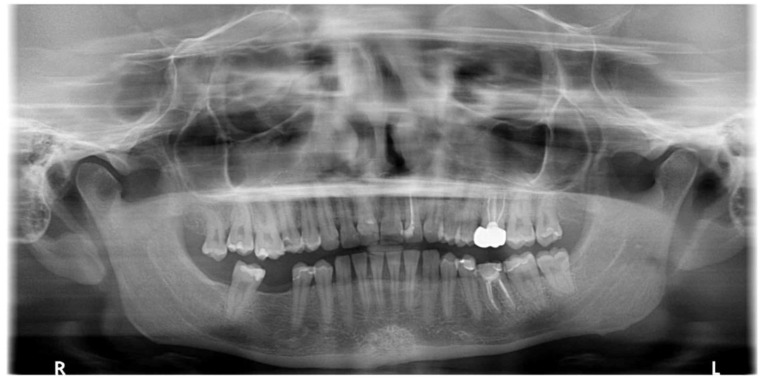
Orthopantomography of the patients before treatment.
